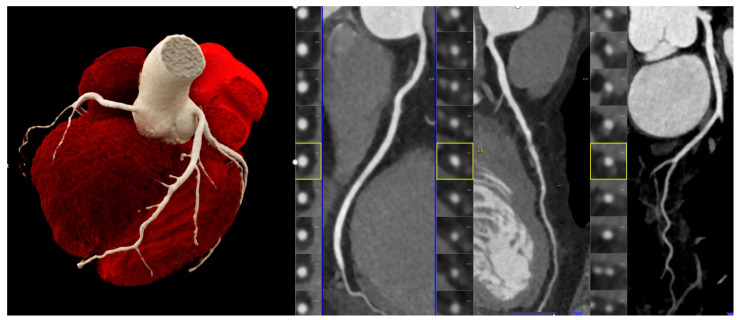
Coronary CTA: Optimal image quality with low image noise and high contrast resolution allows for the reliable exclusion of coronary artery disease. Left: 3D-VRT and right: curved multiplanar reformation (cMPR) of RCA, LAD, and CX arteries show absence of coronary plaque and stenosis.
